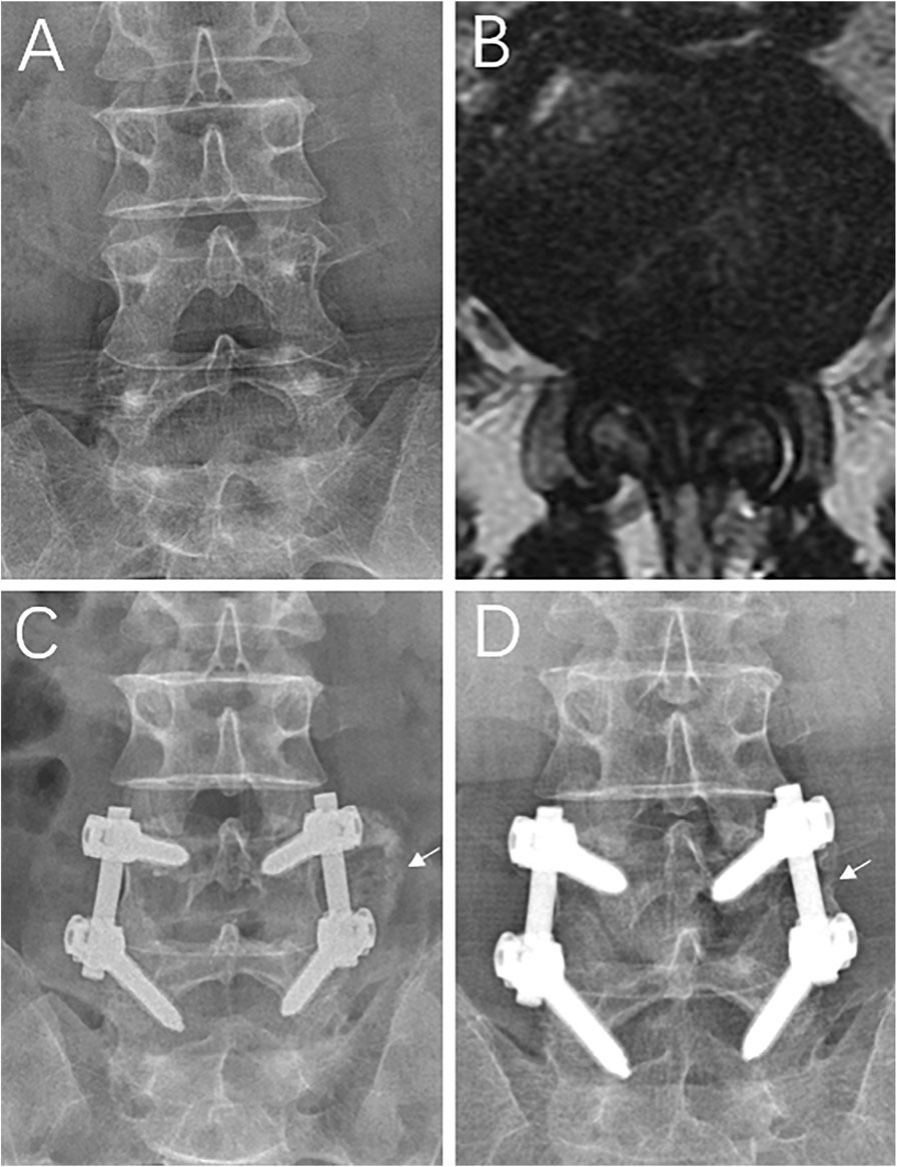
A typical case. A 61-year-old man suffered from L4/5 bilateral spinal canal decompression, fusion, and pedicle screw instrumentation fixation. Before operation, VAS (low back pain) was 5; VAS (radicular pain), 8; JOA, 14; and ODI, 57.6. Two years postoperatively, VAS (low back pain) was 1; VAS score (radicular pain), 1; JOA, 26; and ODI, 10.8. a Preoperative lumbar radiography. b Preoperative lumbar MRI indicating severe stenosis of the L4/5 spinal canal. c Three months after operation, lumbar radiography indicating that the bone graft between the right L4/5 transverse process has been absorbed and that between the left L4/5 transverse process is ossifying. d Two years after operation, lumbar radiography indicating that continuous bony bridge has formed between the left L4/5 transverse process
